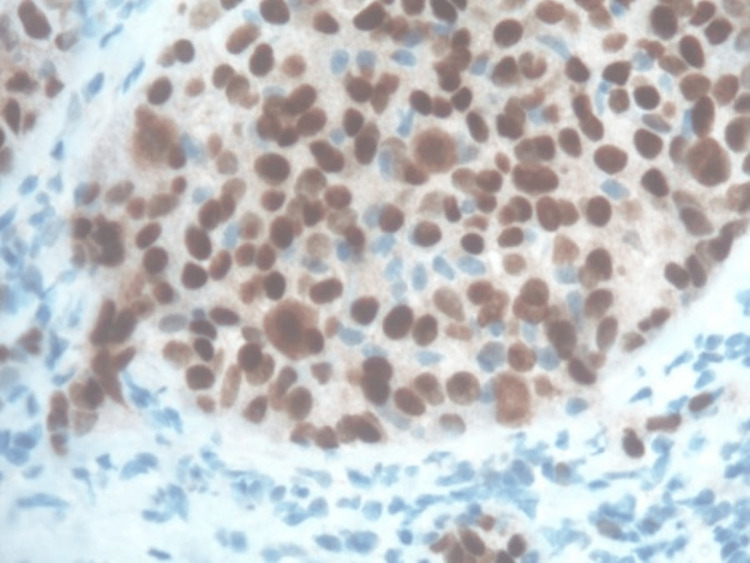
Metastatic clear cell renal cell carcinoma. The neoplastic cells are positive for PAX8, carbonic anhydrase 9, and vimentin and negative for CK7, NKX3.1, PSMA, and RCC. PSMA: prostate-specific membrane antigen; RCC: renal cell carcinoma.
